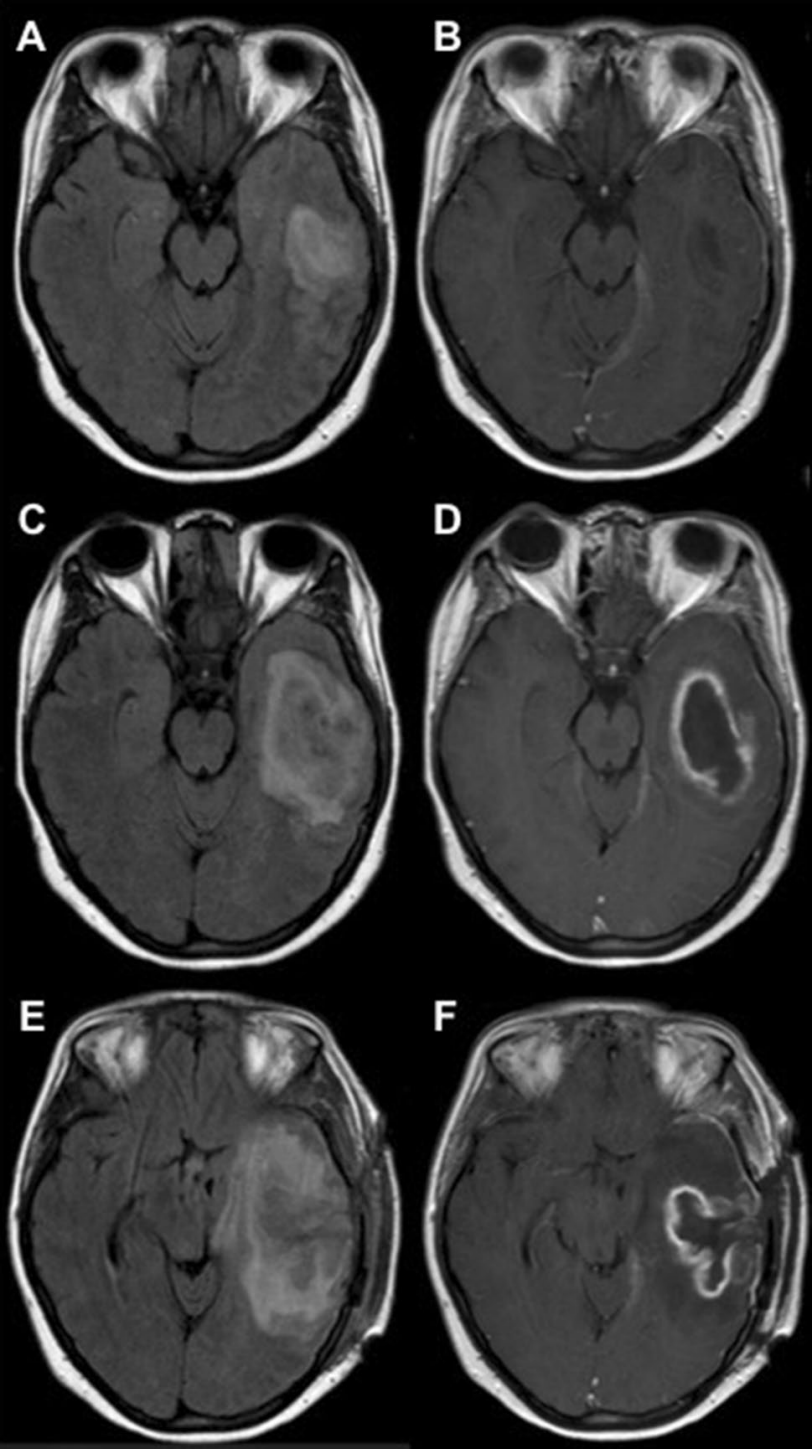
A Early cerebritis, axial fluid attenuated inversion recovery (FLAIR)-weighted MRI. B Early cerebritis, axial contrast-enhanced T1-weighted MRI. C Temporal abscess formation, axial fluid attenuated inversion recovery FLAIR-weighted MRI. D Temporal abscess formation, axial contrast-enhanced T1-weighted MRI. E Abscess relapse with herniation, axial FLAIR-weighted MRI. F Abscess relapse with herniation, axial contrast-enhanced T1-weighted MRI
